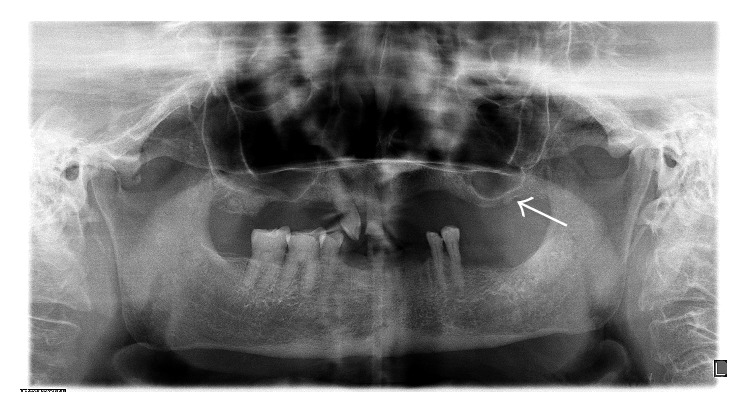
Panoramic radiograph of this patient; root remnant (white arrow) was detected in the left maxillary molar area.
